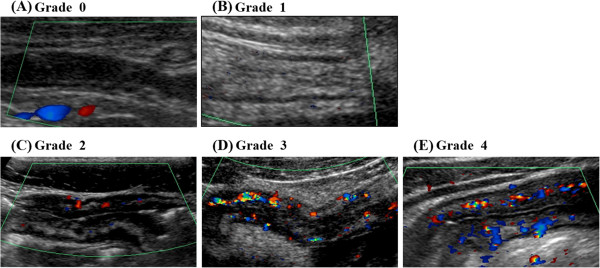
Examples of semiquantitative assessment of bowel wall vascularity using Doppler US according to Limberg score. (A) Grade 0 = no bowel wall thickening, no vascularization; (B) Grade 1 = bowel wall thickening without vascularization; (C) Grade 2 (hypo-flow) = bowel wall thickening with short stretches of vascularity as spots; (D) Grade 3 (hyper-flow) = bowel wall thickening with longer stretches of vascularity; (E) Grade 4 (hyper-flow) = bowel wall thickening with longer stretches of vascularity reaching the mesentery.
